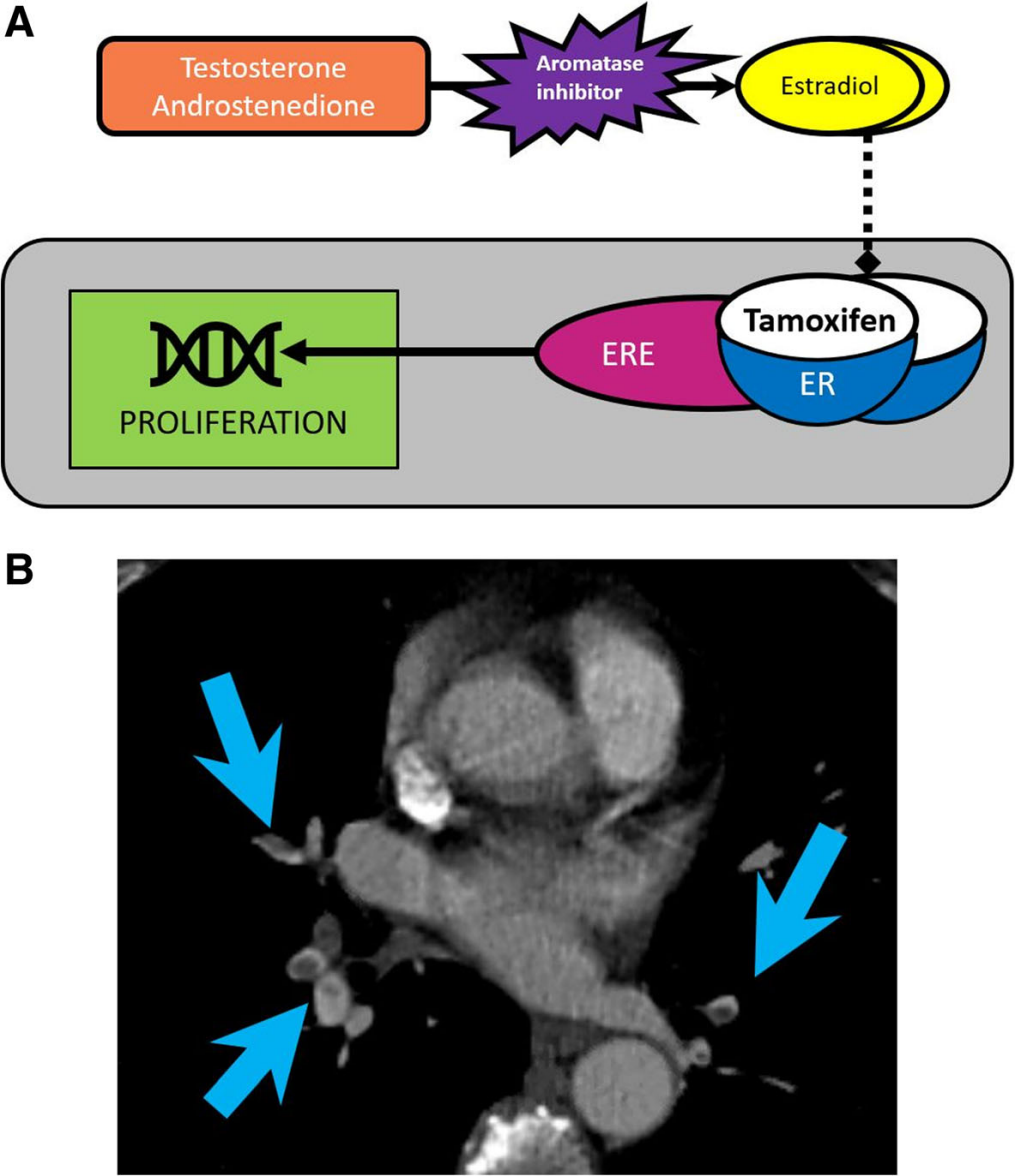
Transcriptional regulation pathway by steroid hormones. a Image showing the role of aromatase inhibitors and tamoxifen in blocking steroid synthesis. Estradiol binds to estrogen receptor (ER), leading to dimerization and binding to estrogen response elements (ERE) activating estrogen-responsive genes leading to proliferation. Tamoxifen competes with estradiol for ER binding aromatase inhibitors decreasing the synthesis of estrogens from their precursors. b Contrast-enhanced CT images of the chest and abdomen in a 72-year-old woman with ER^+^ invasive lobular breast cancer treated with letrozole (aromatase inhibitor) and tamoxifen. CT of the chest shows multiple filling defects in the bilateral segmental pulmonary arteries (arrows), compatible with pulmonary embolism, an adverse event associated with tamoxifen
